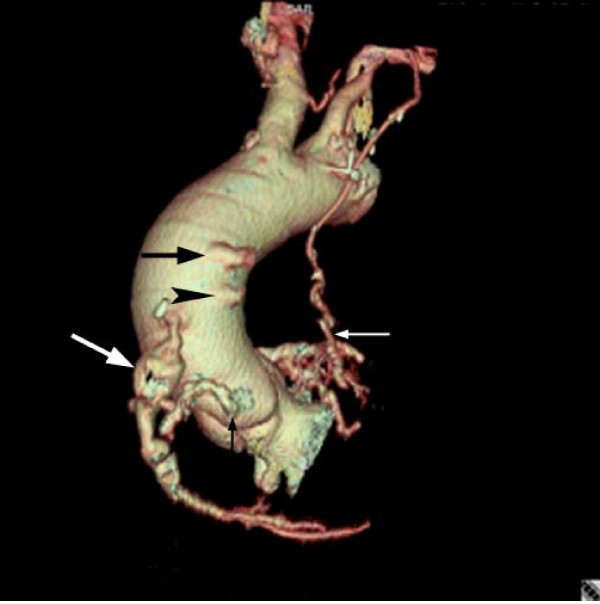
Three dimensional reformatted image from CTA demonstrating the fusiform saphenous vein graft aneurysm (large white arrow) as well as the two thrombosed trunks of the other two saphenous vein grafts (large black arrow and black arrowhead), the native RCA (small black arrow) and the patent LIMA to LAD graft (small white arrow).
